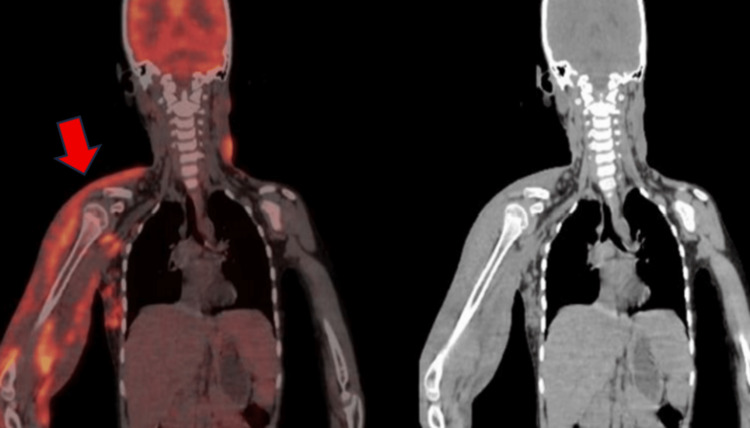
PET-CT demonstrated heterogeneously enhancing, metabolically active diffuse subcutaneous soft-tissue infiltration involving the right upper limb, thoracic wall, right cervical region, and left side of the face PET-CT: positron emission tomography-computed tomography
